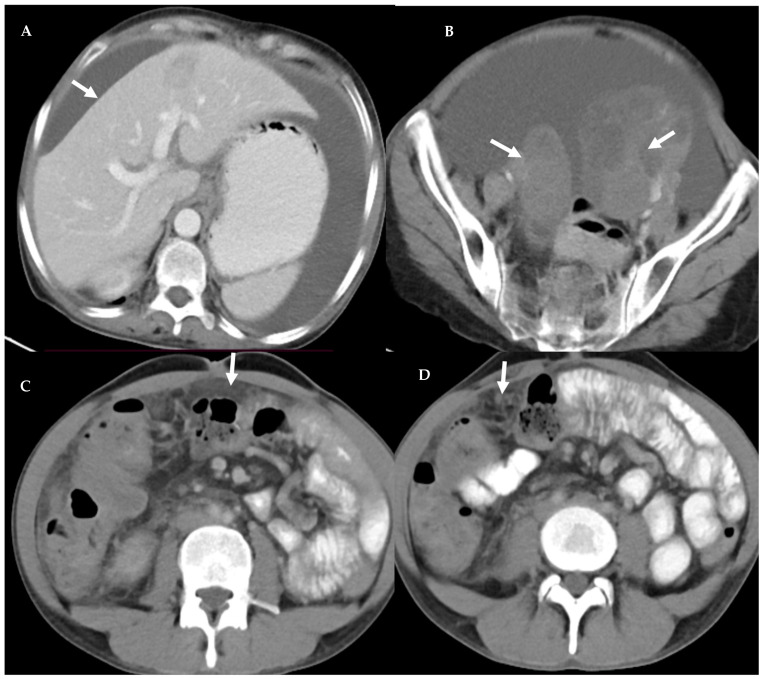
Peritoneal carcinomatosis. Axial contrast enhanced CT images in a 50-year-old lady. Ascites with scalloping of liver surface (arrow) can be seen (A). There are bilateral ovarian masses (arrow) (B). The peritoneum has a smudged appearance (arrow) (C). There are a few omental nodules (arrow) (D).
